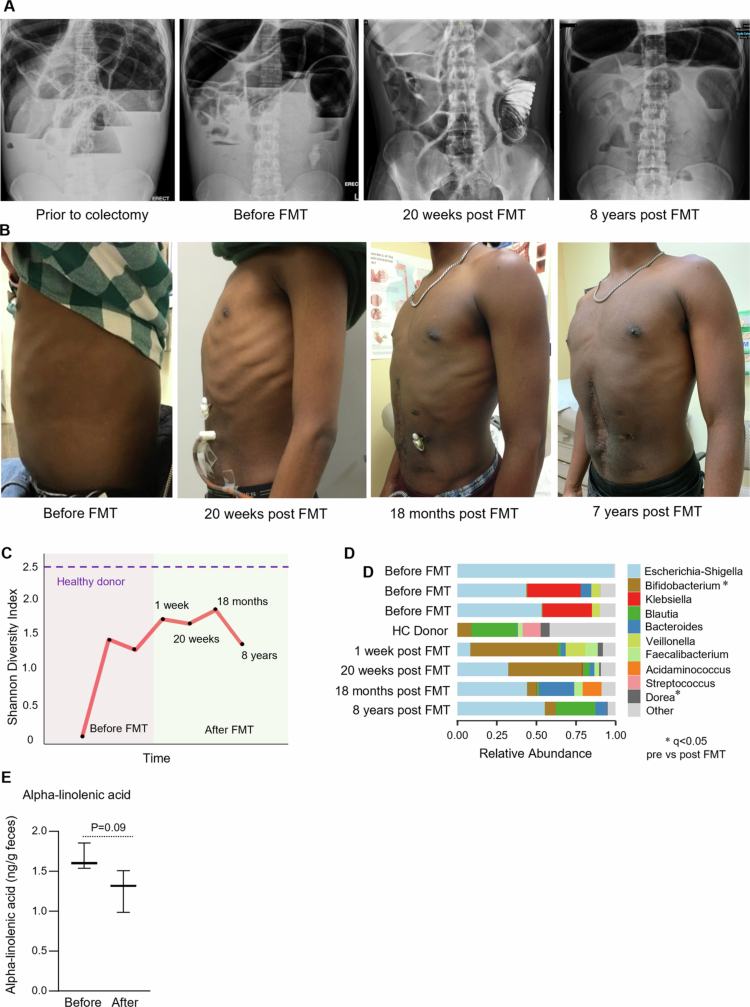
FMT induces normalization of microbiota and longterm clinical improvement in the index CIPO patient. (A) Representative abdominal X-ray images of the index CIPO patient before colectomy, before FMT, and 20 weeks and 8 y post FMT. (B) Representative photographs of the index CIPO patient before FMT, and 20 weeks, 18 months and 7 y post FMT. (C) Longitudinal representation of the alpha diversity, measured as Shannon diversity index, of the microbiome of the index CIPO patient before and after FMT. (D) Taxonomic composition at genus level of the microbiome of the index CIPO patient before and after FMT. The data is shown as relative abundance. *marks significant differences between all pooled samples before FMT and all pooled samples after FMT. *P*-values were calculated with a multivariate regression model using MaAsLin2 67 and a *P-*value < 0.05 after False Discovery Rate (FDR) correction using Benjamini–Hochberg procedure was considered significant. (E) Alpha-linolenic acid was assessed in fecal samples of the index CIPO patient before and after FMT. The data were analyzed with unpaired Mann–Whitney U test. A *P-*value < 0.05 was considered statistically significant.
